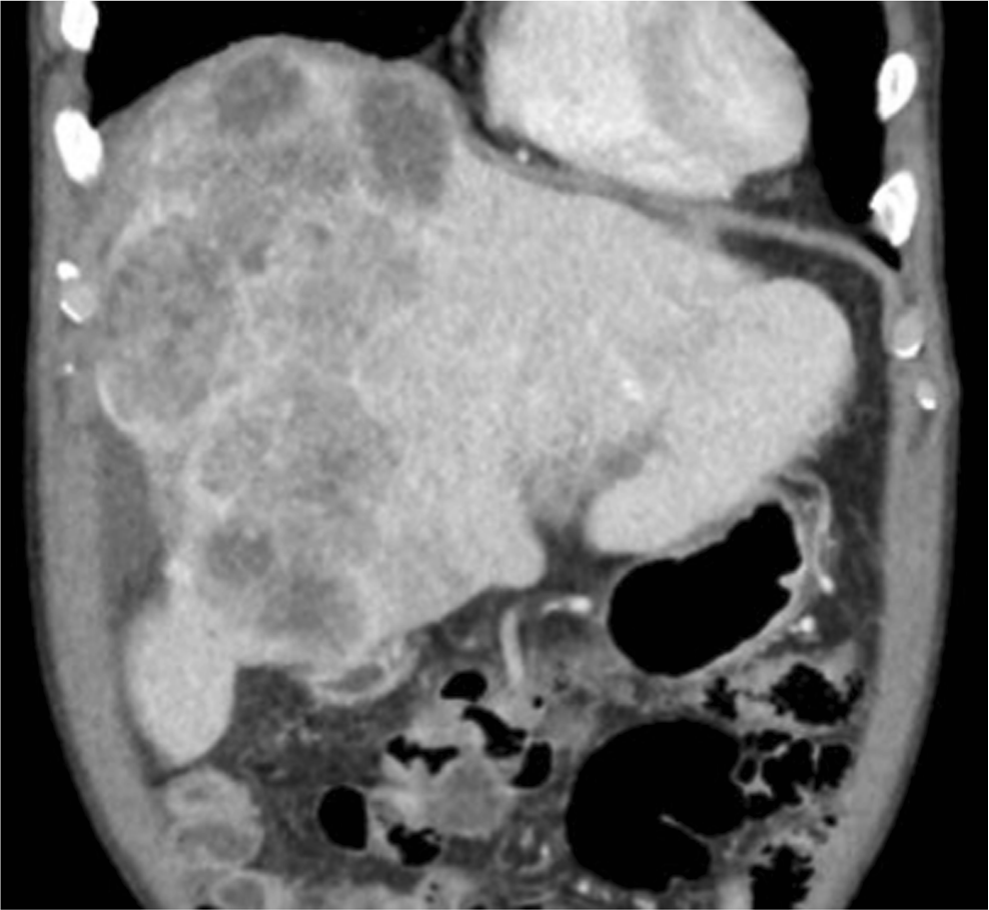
Coronal portal venous phase CT demonstrates washout in the heterogeneous HCC filling much of the right lobe of liver. Capsular retraction is present laterally with ascites filling the void
